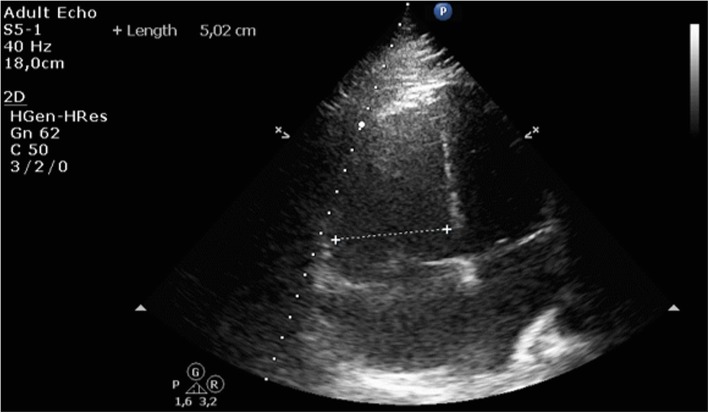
Apical 4-chamber view during echocardiography with right ventricle measurement
